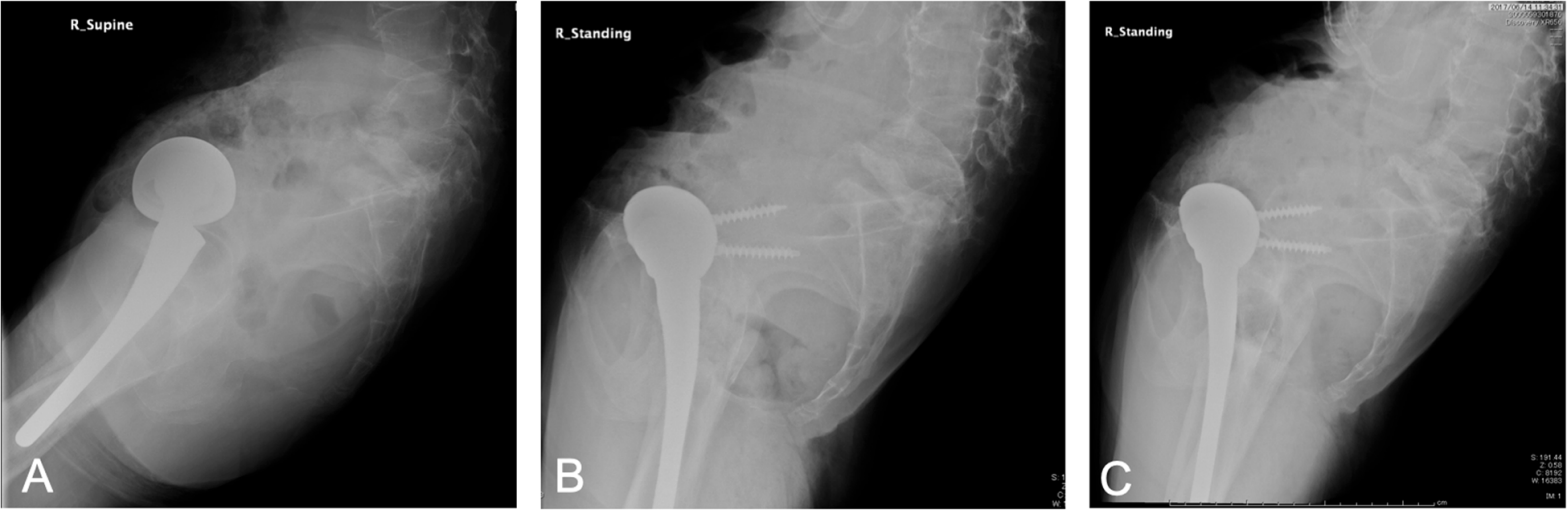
X-ray lateral images of the pelvis, showing the time course of posterior changes in the pelvic tilting angle. Lateral pelvic X-ray images in the supine position preoperatively (a) and in the standing position 1 year (b) and 2 years (c) postoperatively
